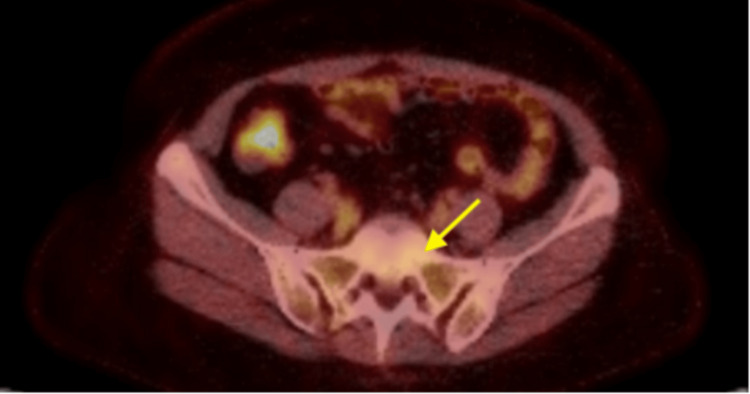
PET-CT scan demonstrating multiple small sclerotic foci within multilevel vertebral bodies and sacrum.
